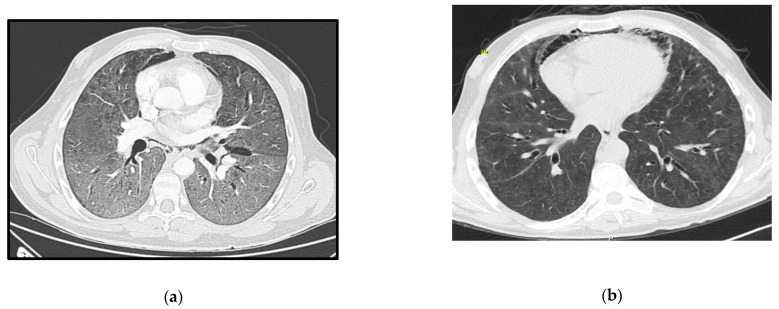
Case 1 thoracic CT-scan at diagnosis (a) and follow-up (b).
